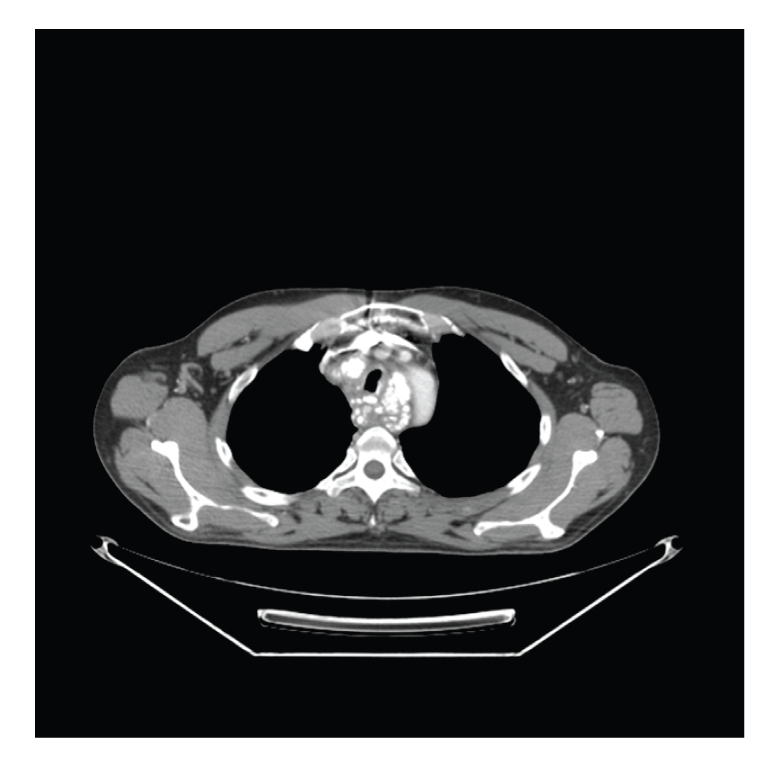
Lobulated, calcified esophageal mass at T4 level, as seen on CT scan. The mass almost completely surrounds the trachea and essentially obliterates the esophagus. Extensive calcification of a soft tissue mass and its presumed metastases should make one consider EOO as a possible diagnosis.
